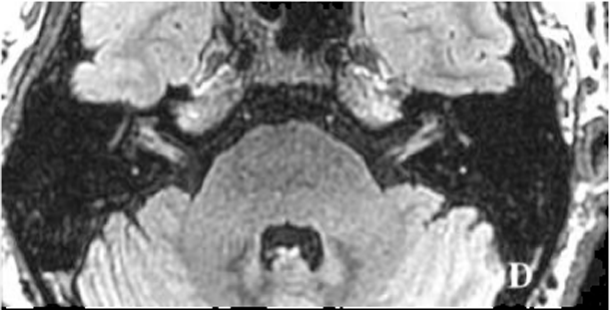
In December 2020, the left vestibular filling defect became larger under gadolinium angiography in the 3D-T2-FLAIR sequence, which was a sign of EH (D).
